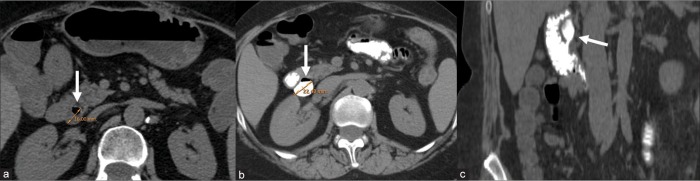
A 64-year-old woman with a history of recurrent postprandial epigastric pain. After US examination, which demonstrated common bile duct dilatation in the absence of lithiasis nuclei, the patient underwent unenhanced computed tomography (a), which demonstrated a periampullary duodenal diverticulum (white arrow) with a strict neck. Oral contrast was administered to better identify diverticulum, which was increased in maximum diameter (b) from 16 mm to 22 mm. (c) Coronal reconstruction shows periampullary duodenal diverticulum with a cranial ventral growth/expansion.
